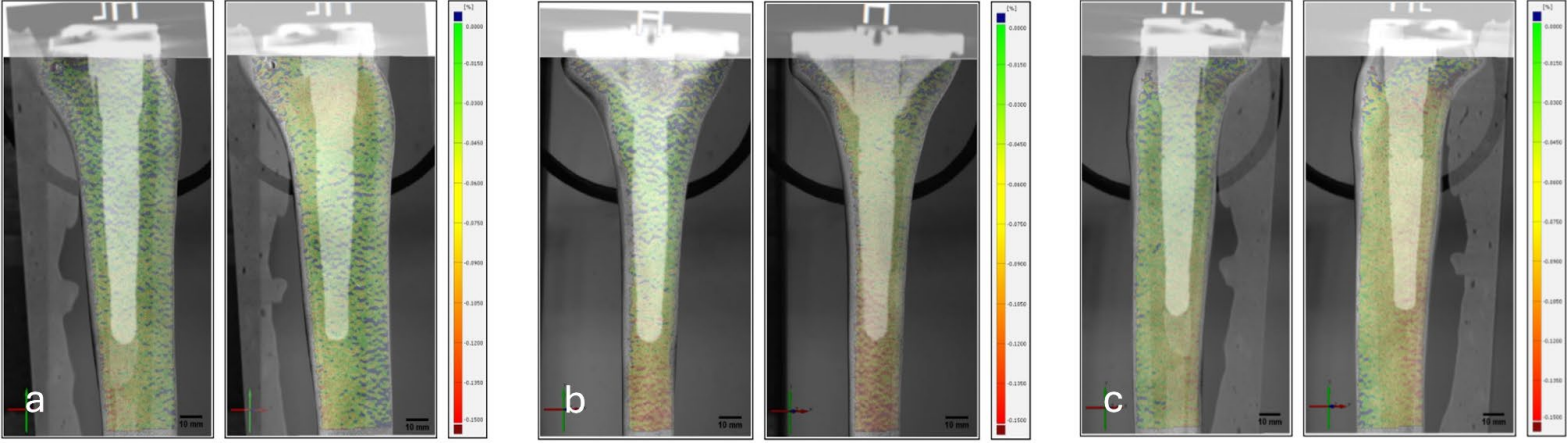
Displayed field of strains εy [%] by CT scans from ventromedial (a), dorsal (b) and ventrolateral (c)
